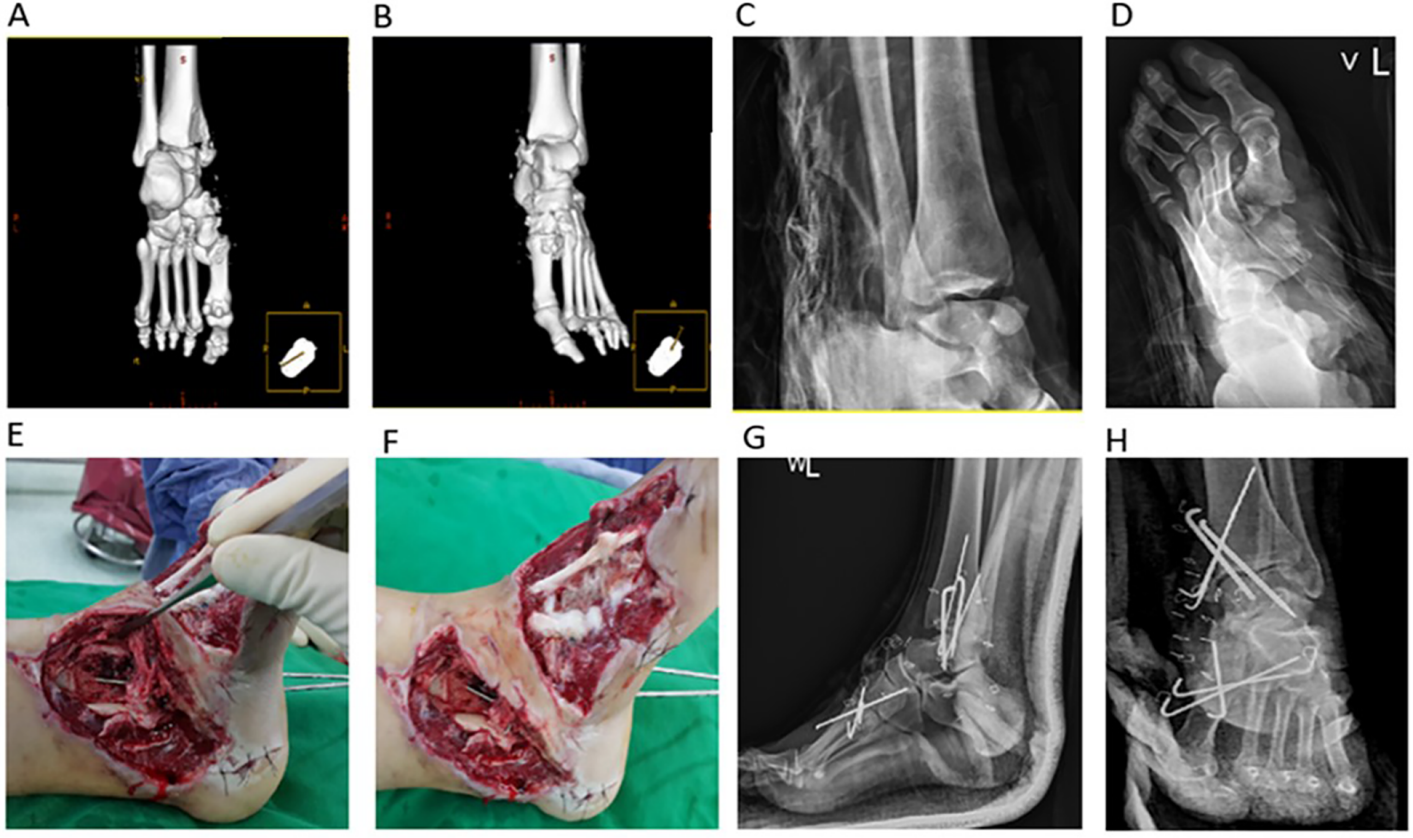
(A–D) Preoperative images (E,F) intraoperative images (G,H) postoperative images. Initial radiographs and CT-based 3D bone reconstruction of the left ankle show a large bony defect at the MM and midfoot fractures (A–D). During surgery, devitalized tissues were removed, thereby revealing significant bone loss, deltoid ligament injury, and soft tissue defects in the MM and foot. The MM remnant was stabilized with two 1.8 mm Kirschner wires. Two 2.0 mm Kirschner wires were used to address the tibiotalar dislocation (E,F). Postoperative radiographs show two 1.8 mm Kirschner wires stabilizing the MM, three 1.8 mm Kirschner wires for the 1st and 2nd tarsometatarsal joints (i.e., Lisfranc injury), and two 2.0 mm Kirschner wires for the tibiotalar dislocation (G,H). CT, computed tomography; 3D, three-dimensional; MM, medial malleolus.
